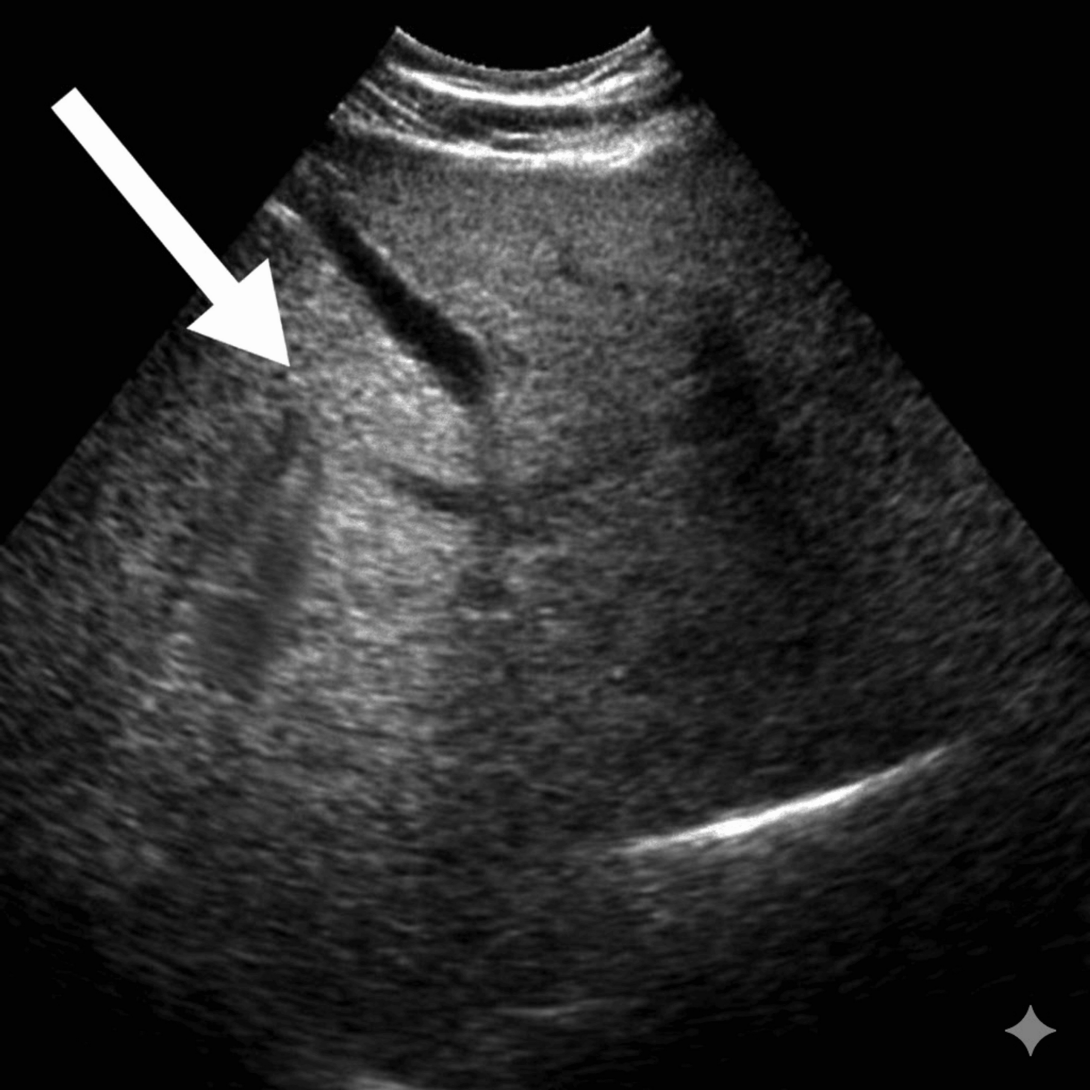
Ultrasound shows increased hepatic echogenicity with poor visualization of the intrahepatic vessel walls (arrow), producing a diffusely bright liver consistent with hepatic steatosis (fatty liver).
